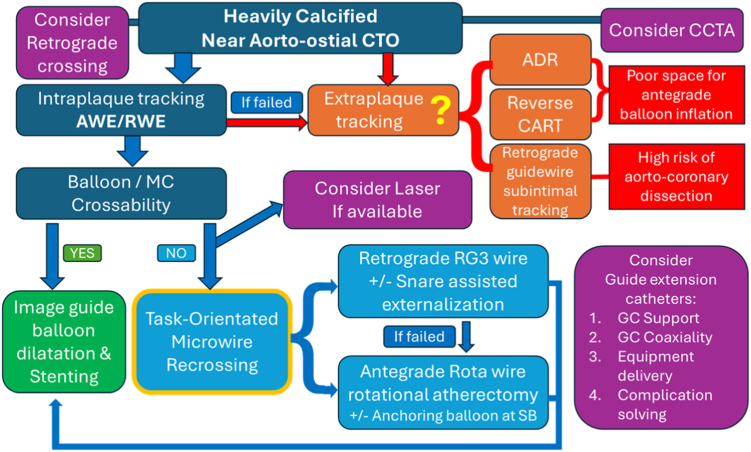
The algorithm for heavily calcified near aorto-ostial chronic total occlusion (CTO) crossing. Aorto-ostial CTO recanalization is challenged by complex anatomy and dense calcification, often precluding extraplaque strategies. A task-oriented direct wiring crossing the created microfractures offers a viable solution. This includes retrograde RG3 externalization with snare assistance for support, and antegrade RotaWire delivery for rotational atherectomy, often facilitated by side branch balloon anchoring. ADR, antegrade dissection reentry; AWE, antegrade wire escalation; CCTA, coronary computed tomography angiography; GC, guide catheter; MC, microcatheter; reverse CART, reverse controlled antegrade and retrograde tracking; RWE, retrograde wire escalation; SB, side branch.
